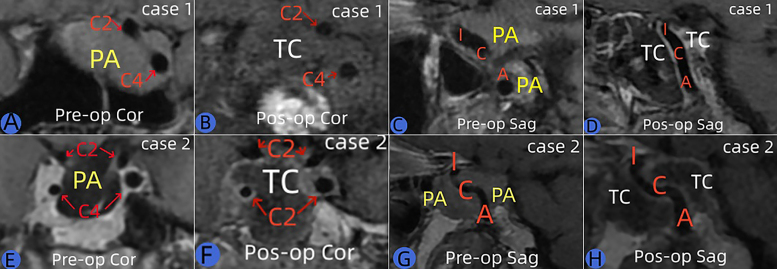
Preoperative and postoperative magnetic resonance imaging for 3 patients who underwent tumor excision combined with resection of the cavernous sinus medial wall. (A–D) Case 1. (E–H) Case 2. C2 = C2 segment of the ICA, C4 = C4 segment of the ICA, Cor = coronal view, ICA = internal carotid artery, Pos-op = postoperative, Pre-op = preoperative, PA = pituitary adenoma, Sag = sagittal view, TC = tumor cavity.
